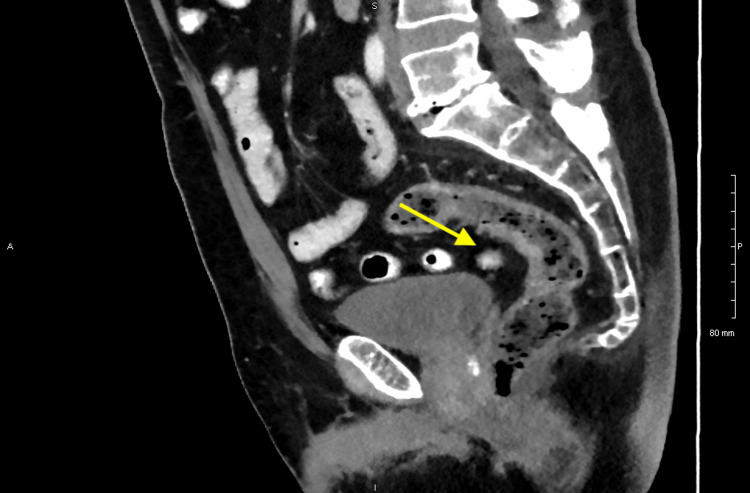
Computed tomography (CT) scan of the abdomen showing a necrotic perirectal lymph node.
